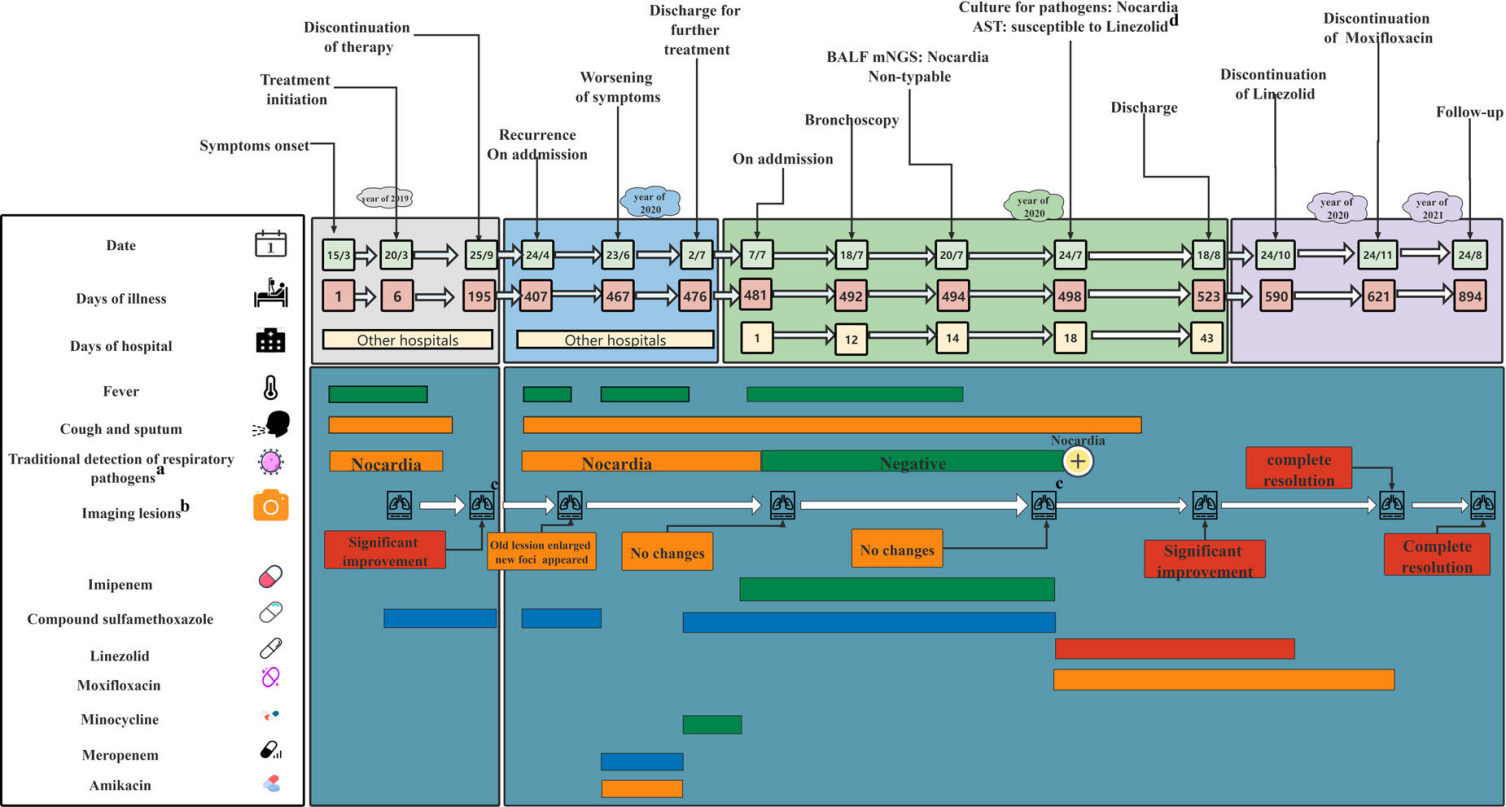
Timeline of the patient’s clinical course. Clinical course of the patient’s symptoms and treatment according to the day of illness and day of hospitalization from March 15, 2019, to August 24, 2021. BALF, bronchoalveolar lavage fluid; mNGS, metagenomic next-generation sequencing; AST, antimicrobial susceptibility testing. a: Detection of viruses, fungi, tuberculosis bacteria and other bacteria. Additional details are provided in Supplementary Table 6. b: The patient underwent CT examinations on March 20, 2019; September 20, 2019; May 27, 2020; July 8, 2020; July 21, 2020; August 17, 2020; December 21, 2020; and August 24, 2021. Each CT examination was compared with the previous examination to assess whether the lesions had improved. The CT examinations performed on September 20, 2019, and July 21, 2020, are not shown in this article, and the others can be found in Figure 2. c: Data are not shown. d: AST results showed that GZ2020T is susceptible to linezolid and possibly to moxifloxacin.
